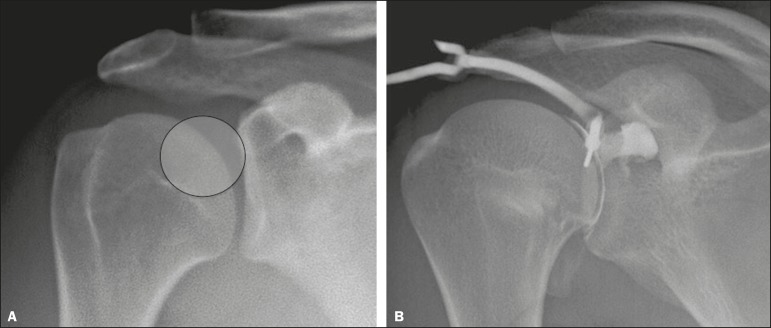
Digital radiograph of the shoulder (A) showing the ideal
